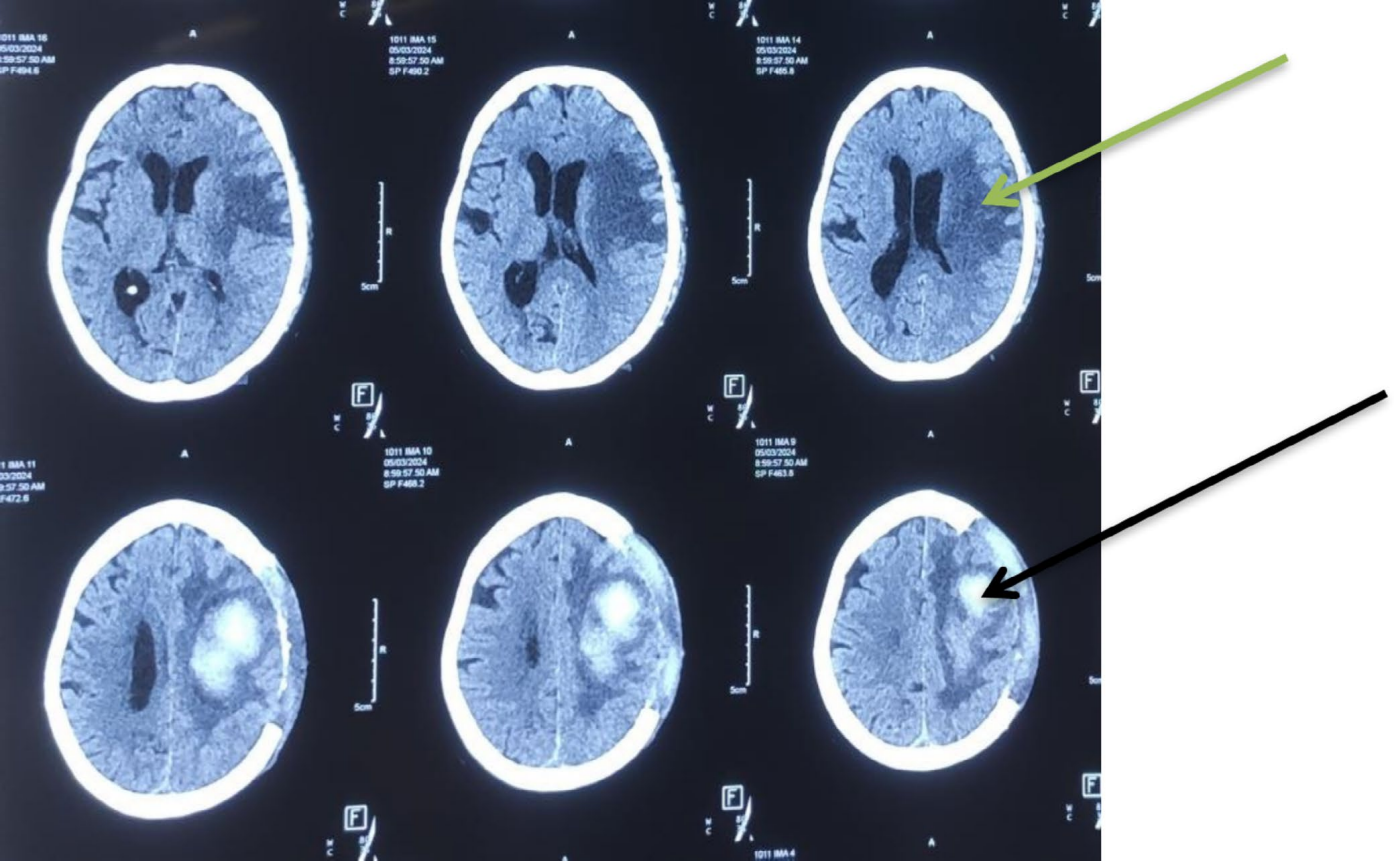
Post‐left fronto‐temporo‐parietal decompressive craniectomy plain CT head showing craniectomy changes of left side with cerebral edema (green arrow) surrounding the ICH core (black arrow) without any obvious midline shift.
